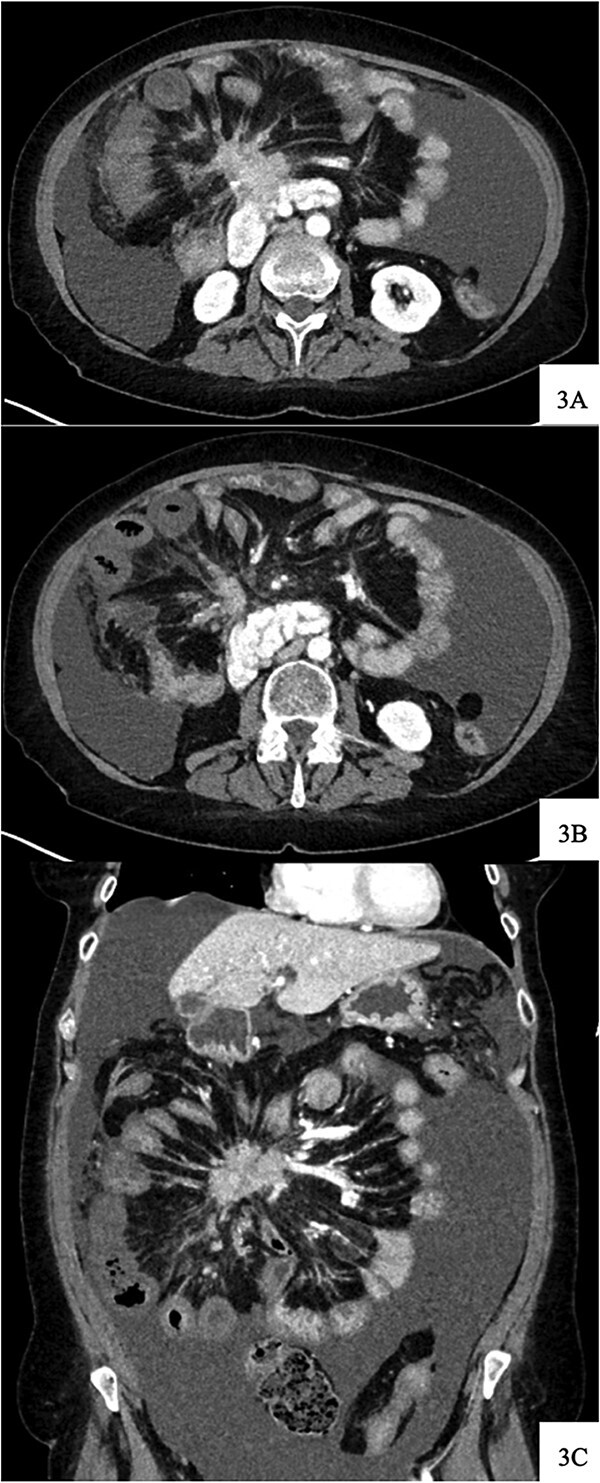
Contrast-enhanced axial CT image at time of presentation shows spiculated central mesenteric mass severe narrowing of the SMV with probably occlusion and encasement of the SMA with possible occlusion (A) associated with decreased enhancement and bowel wall thickening of several small bowel loops within the right lower quadrant (B). Coronal reformatting demonstrates the relationship of the mesenteric mass and affected small bowel (C).
